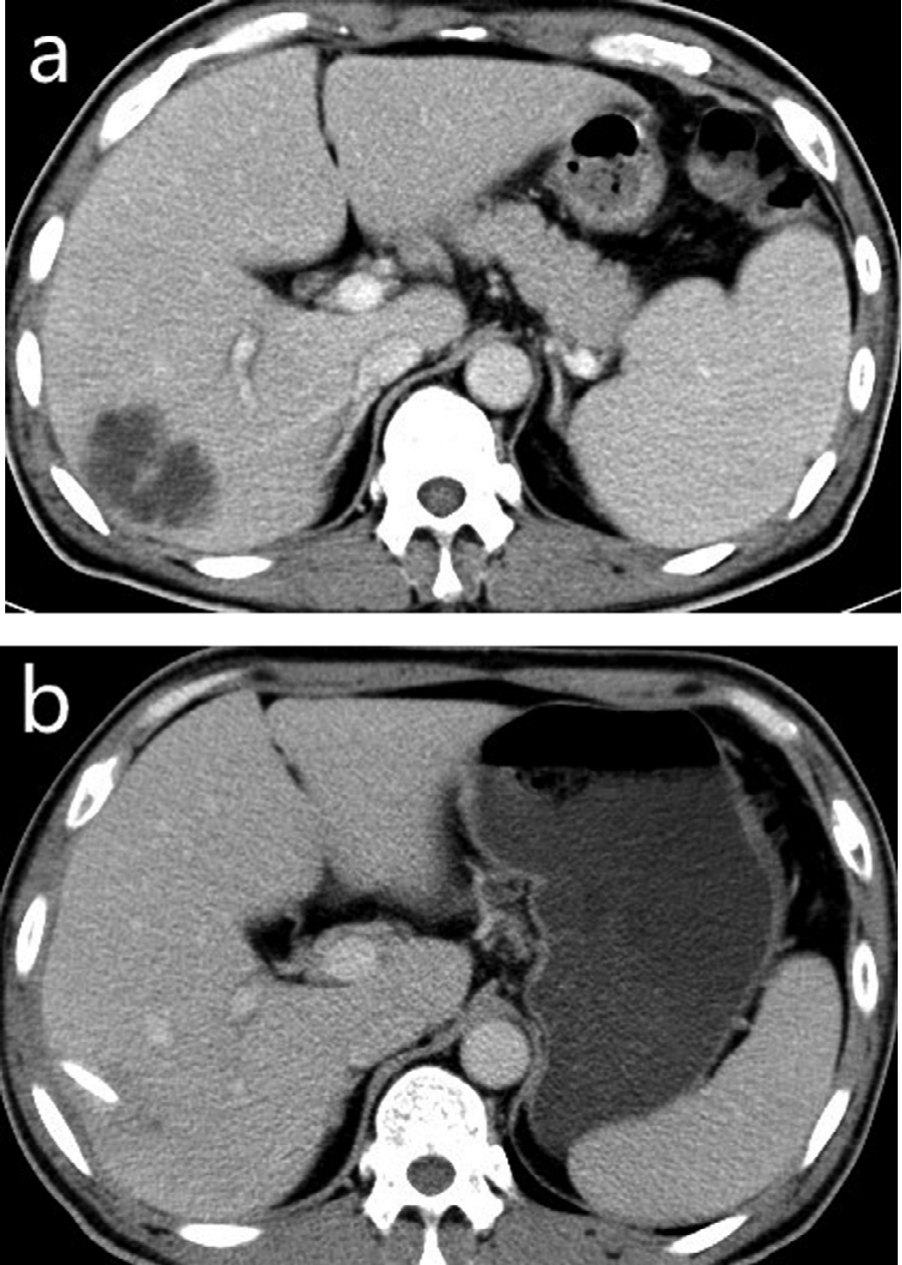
In a 53-year-old liver abscess male without diabetes mellitus, the contrast-enhanced portal-venous phase CT images depict the preoperative liver abscess cavity (a) and the shrinkage of it (b), and no pus drainage can be found 18 days after the CT-guided interventional therapy.
